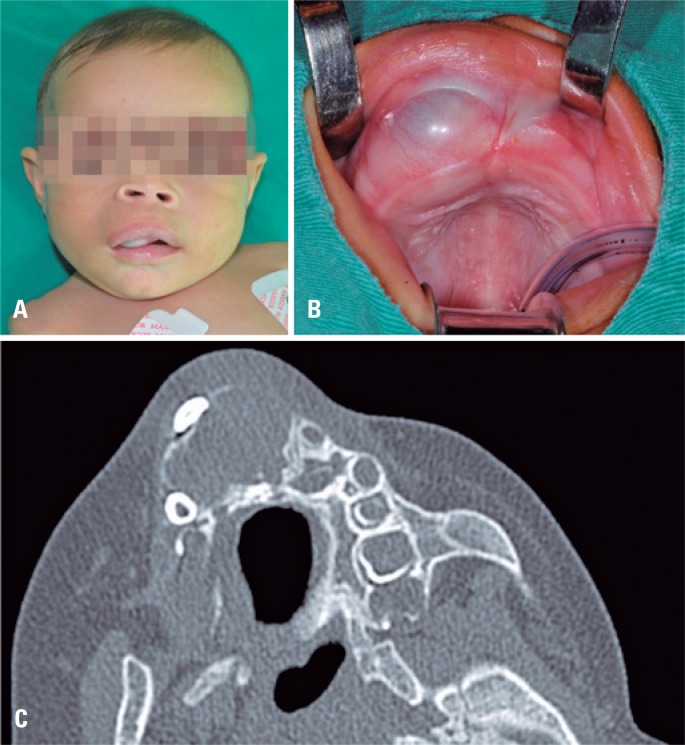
Clinical and imaginological findings of the patient. (A) Extraoral examination showing elevation of right upper lip and paranasal region. (B) Intraoral aspect of the melanotic neuroectodermal tumor of infancy revealing an expansive mass covered by intact mucosa. (C) Computed tomography scan showing a well-defined unilocular, osteolytic lesion causing expansion and destruction of the buccal cortical bone and involvement of the primary deciduous incisive tooth
